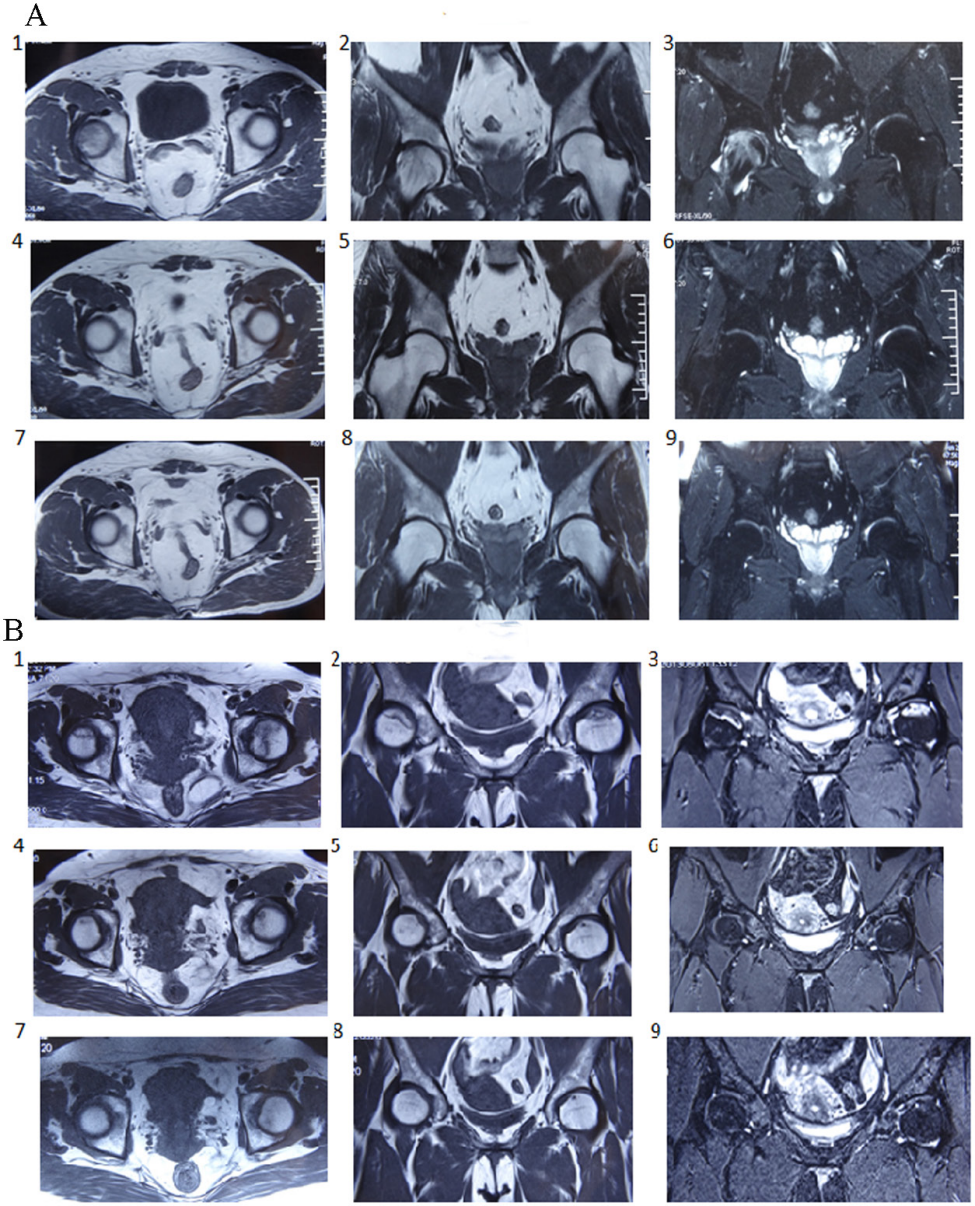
Representative case of osteonecrosis of the femoral head (ONFH) evaluated by magnetic resonance imaging (MRI) (A) 54-year-old male patient, alcoholic (250 ml of alcohol per day). 1 - 3: There were abnormal signals on the right side of osteochondritis of the femoral head and an extensive area of abnormal signals under the perichondrium. 4 - 6: After treatment with the bone-strengthening pill (BSP) for 6 months, the abnormal signals disappeared and the patient resumed normal work. 7 - 9: The MRI image had no abnormalities at 1-year follow-up. (B) 49-year-old female patient, steroid administration 5 years ago and ONFH for 2 years. 1 - 3: MRI showed slight collapse of the bilateral femoral head. There were large and irregular long T1 and long T2 signals on the anterior superior segment of the bilateral femoral head. STIR revealed that the necrotic lesions exhibited a ladder-like, high, mixed, uneven abnormal signal, disappearance of cartilage signal especially on the left side. 4 - 6: The abnormal signals decreased and the patient could undertake light work after treatment for 6 months. 7 - 9: At 1-year follow up after treatment, the MRI was normal and patient could work as usual.
